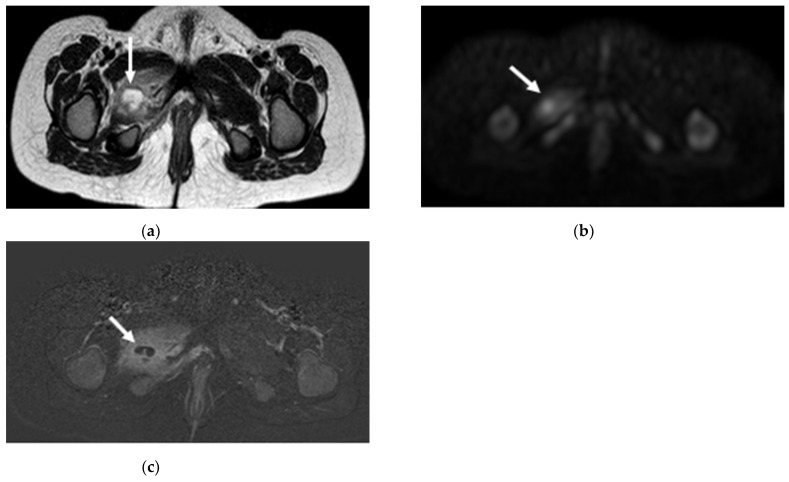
Pyomyositis of the obturator externus muscle in a 3-year-old child with a history of a fall from the bicycle. (a) Axial T2-weighted turbo spin echo (TSE) image shows the abscess (arrow) as an hyperintense fluid collection with hypointense wall. The abscess appears hyperintense (arrow) on (b) diffusion-weighted imaging (DWI) and hypointense with a thin hyperintense rim (arrow) on (c) axial post-contrast image.
